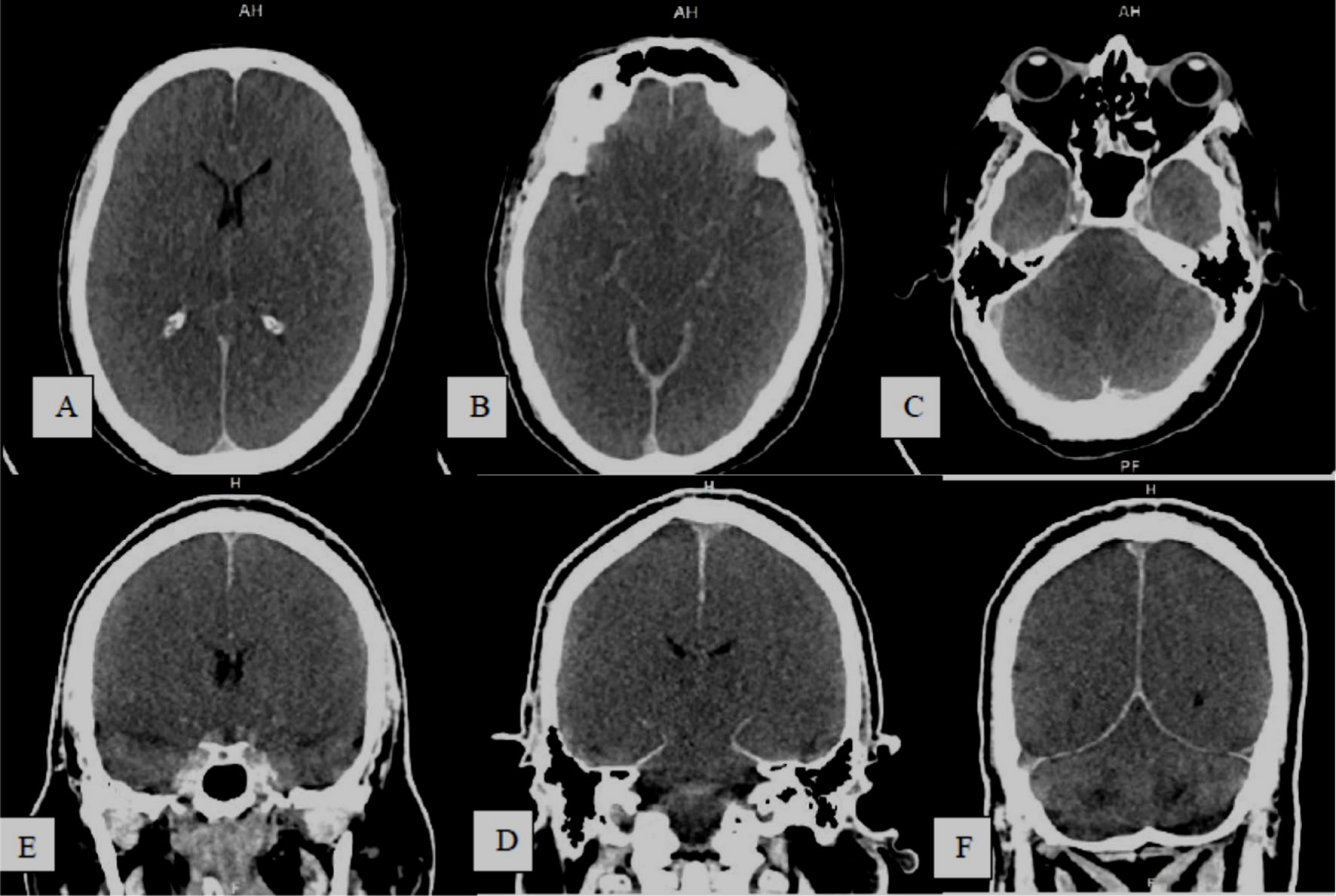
60-year-old COVID-19 male patient with impaired level of consciousness, A–C serial axial CT brain study without contrast, D–F serial coronal reformatting images, showing poor gray/white matter differentiation with diffuse brain parenchyma hypodensity reflecting *diffuse*
